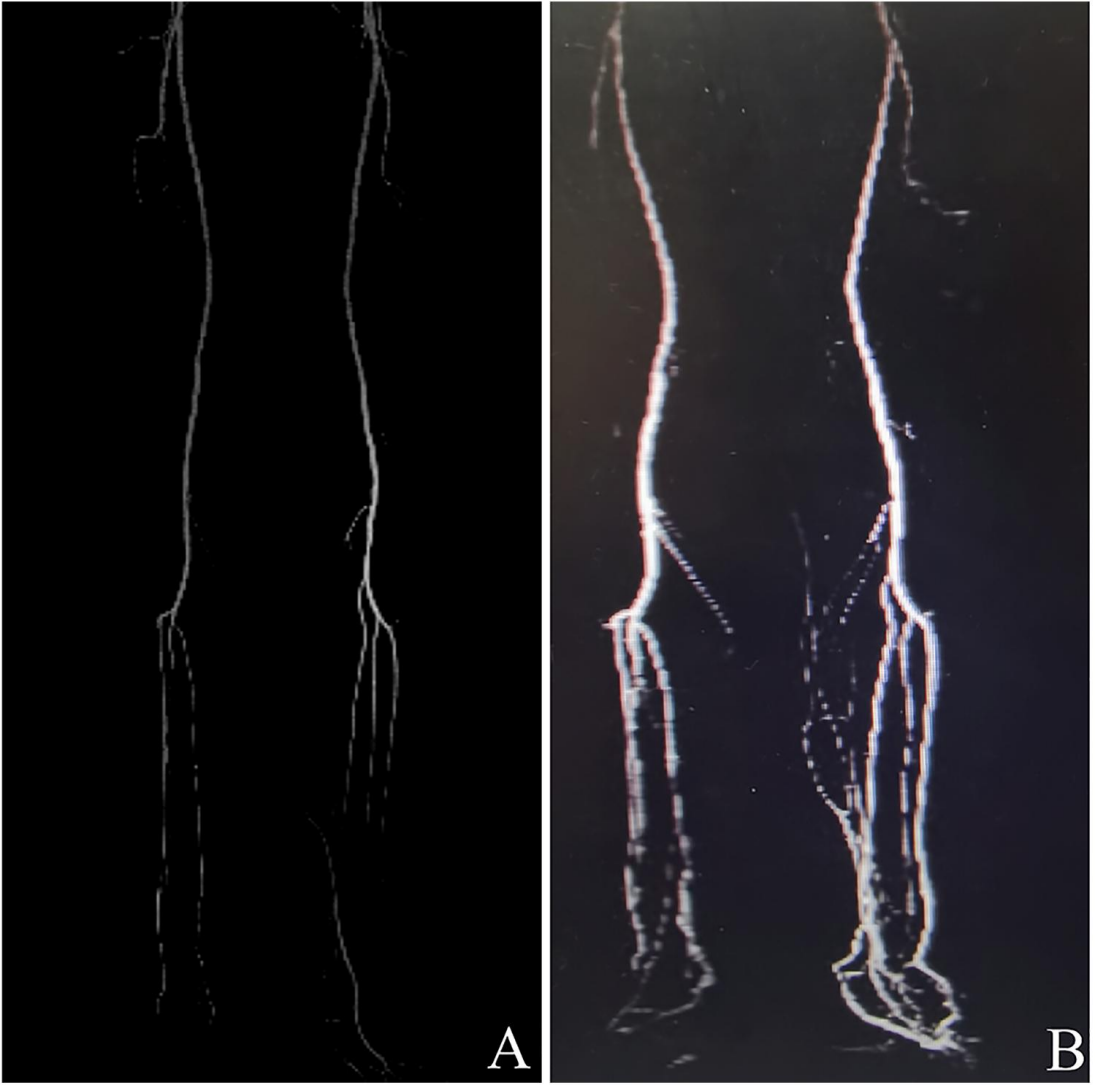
Noninvasive CTA result of a patient who underwent TTT surgery. (A) Before PTA surgery, the CTA analysis reveals femoral artery stenosis, infra-popliteal artery stenosis, and occlusion. (B) One year after TTT surgery, CTA demonstrates a substantial improvement in vascularization and hemadostenosis at both low extremities, including smoother and thicker arteries, increased vessel numbers, and established collateral circulation. CTA, computed tomographic angiography; TTT, tibial cortex transverse transport; PTA, percutaneous transluminal angioplasty.
